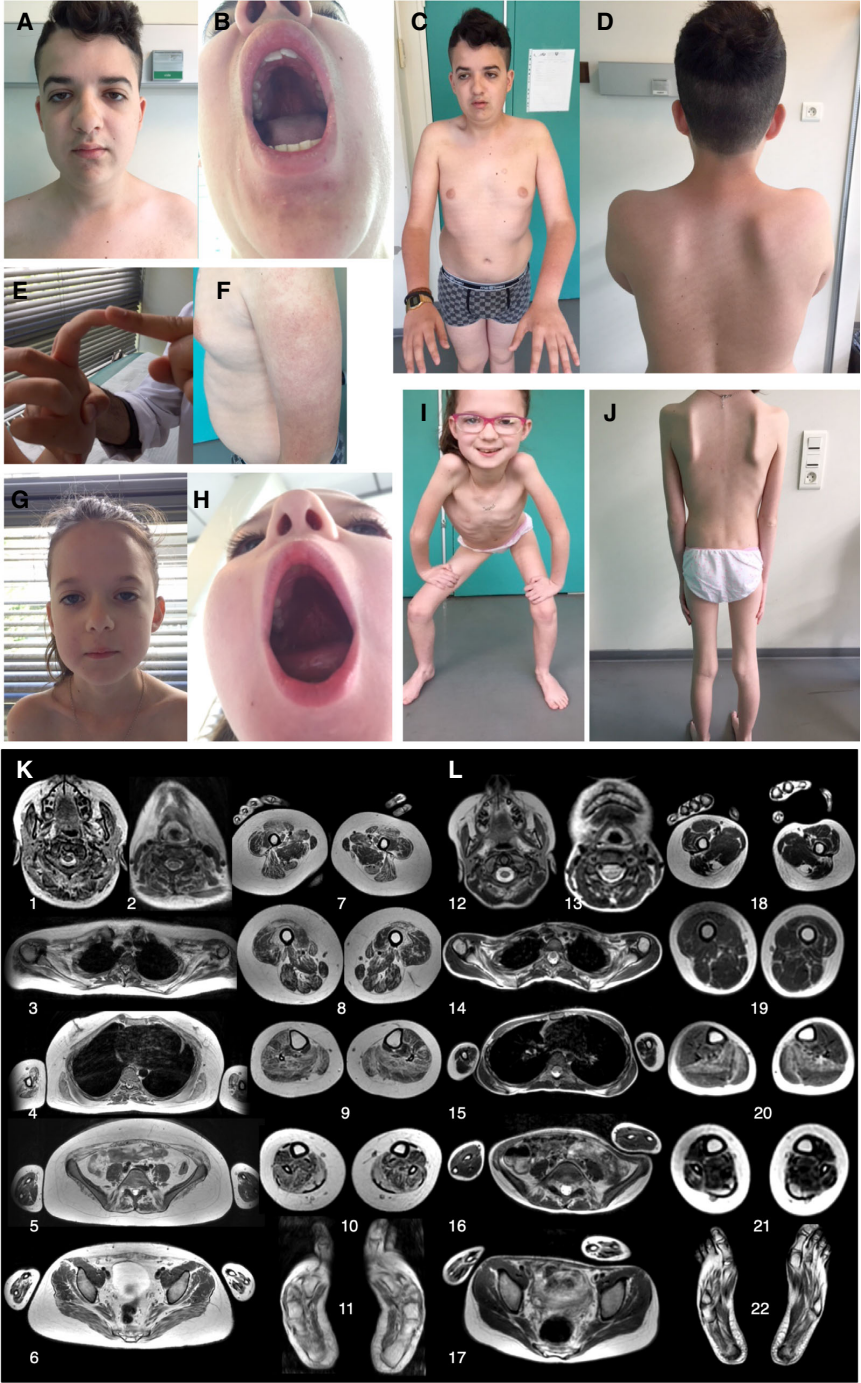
Clinical presentation and MRI features. (A–F) P1’s clinical features. (A) Mildly elongated face with bilateral ptosis and low‐set ears. (B) High‐arched palate. (C) Deficit in arm elevation (45°). (D) Scapular winging. (E) Distal joint hyperlaxity. (F) Prominent hyperkeratosis pilaris. (G–J) P2’s clinical features. (G) Mild ptosis without other facial dysmorphisms. (H) High‐arched palate. (I) Pelvic muscle proximal weakness; the patient cannot squat. Note the extremely thin muscle bulk. (J) Thin muscle bulk, mild scapular winging. (K and L) Three‐dimensional volume rendering reconstruction of MRI sequences in P1 (K1‐11) and P2 (L12‐22) with anterior, lateral and posterior views. These views allow a global analysis of the patients’ phenotype. Selection of 11 slices among the 350 mm thick, contiguous axial slices from head to toe; DIXON T2 (IDEAL T2) in‐phase images. P1 is older and the disease is more advanced with more fat infiltration and atrophy compared with P2. The distribution of involved muscles is similar but less pronounced in P2. Only one muscle is more fatty ‐infiltrated in P2. The upper part of the semi‐tendinous muscle is as white as subcutaneous fat in P1 (K‐7). The sternocleidomastoid, ileo‐psoas, *gracilis*, *adductor magnus* and common toe extensors are less affected by the disease.
